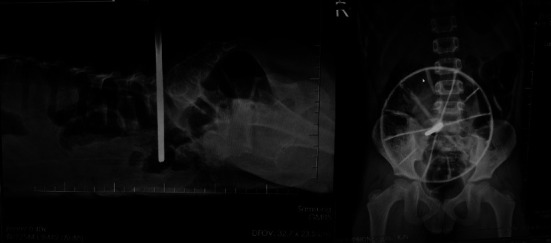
Radiographic imaging of the patient shows a radiopaque metallic rod and wheel seen at the right hemiabdomen immediately lateral to the L5 vertebrae with no apparent bone involvement at the time of the exam.
